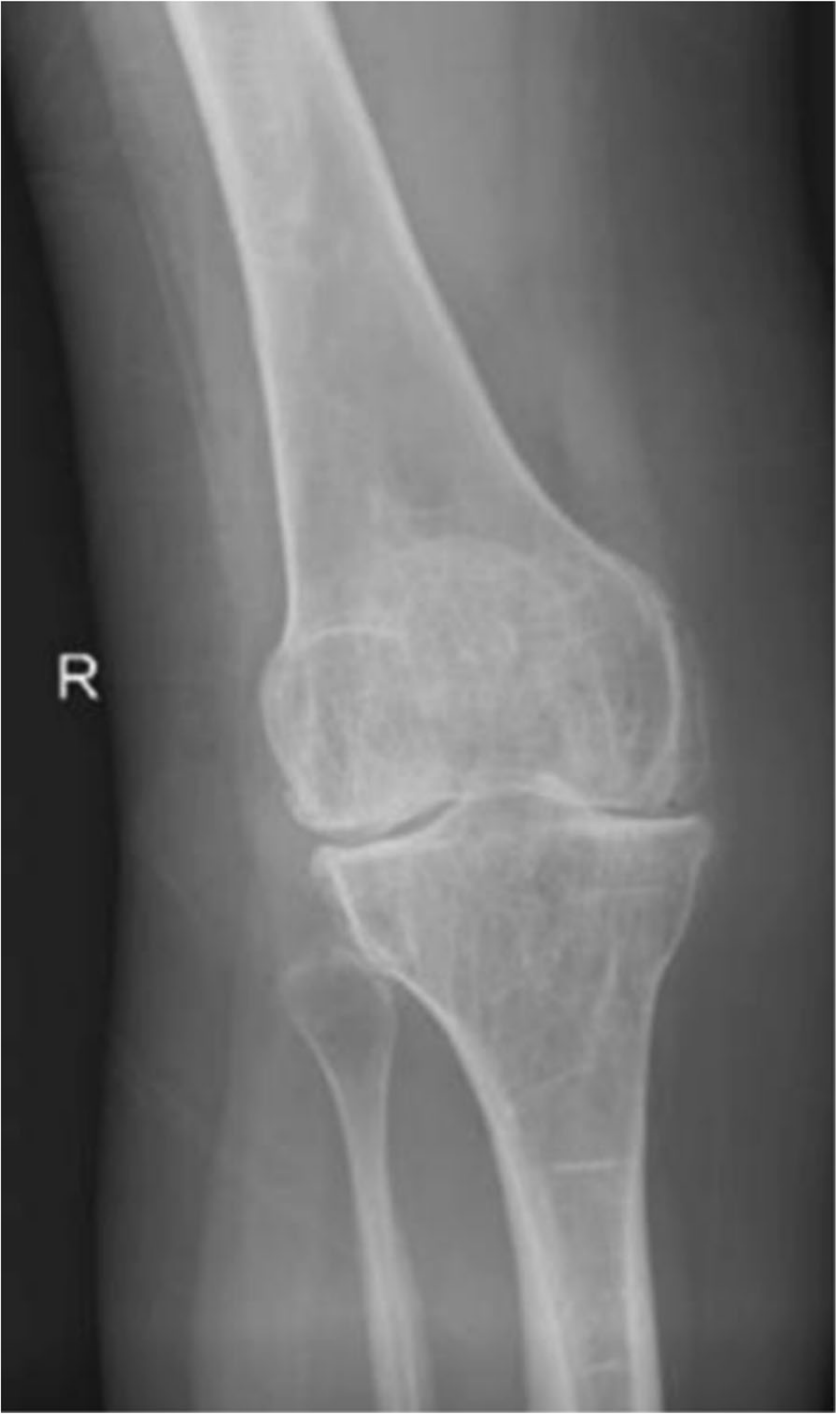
A preoperative AP radiograph demonstrating the degenerative changes in the knee
